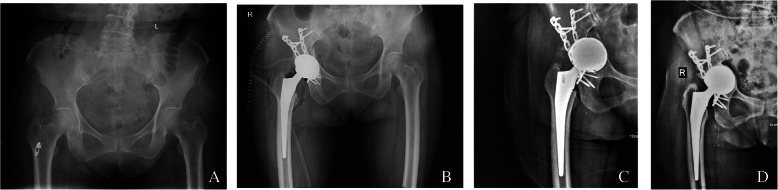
a Patient 5,Preoperative x-ray. b X-ray after operation for 3 days, posterior column fracture, internal fixation with steel plate screws. c X-ray 2 months after operation. d X-ray 3 years after operation, the component is stable
